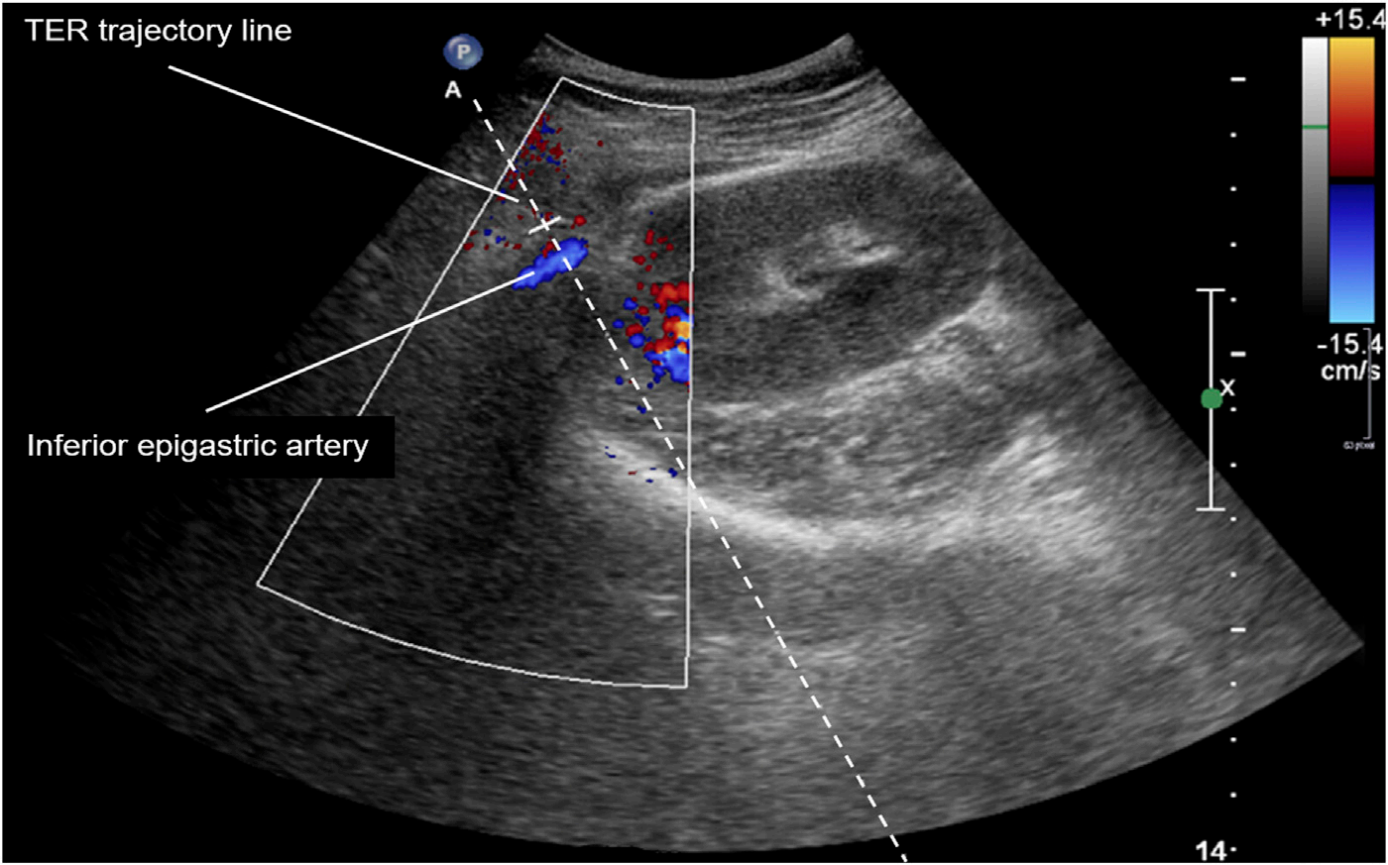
Color-duplex ultrasound image of right iliac kidney allograft and dislocated inferior epigastric artery. TER, tangential, extraperitoneal, retrorenal.
